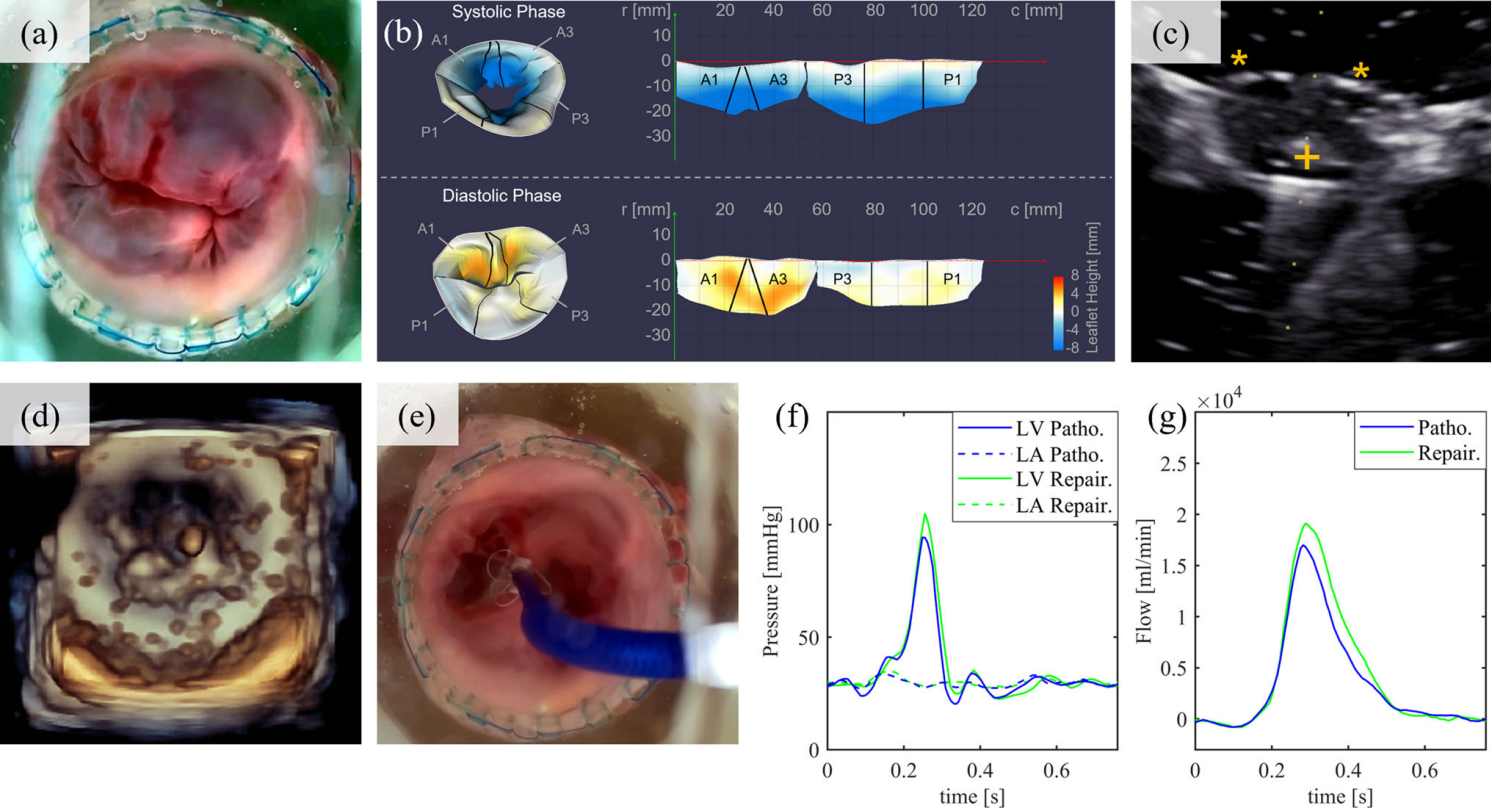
a Ex-vivo valve B in dilated annulus pathology. b 3D-view (left) and unrolled 2D-view (right) of the valve during systolic (top) and diastolic (bottom) phase. c, f B-mode (c) and 3D-ultrasound image (d) in repaired condition. e after TEER. f, g Pressure (f) and Flow (g) graphs. C, annulus circumference; r, radial distance to the annulus plane; A1–3, anterior leaflets; P1–3, posterior leaflets; + , clip; *, leaflet; LV, left ventricle; Patho., pathological condition LA, left atrium; Repair., repaired condition
